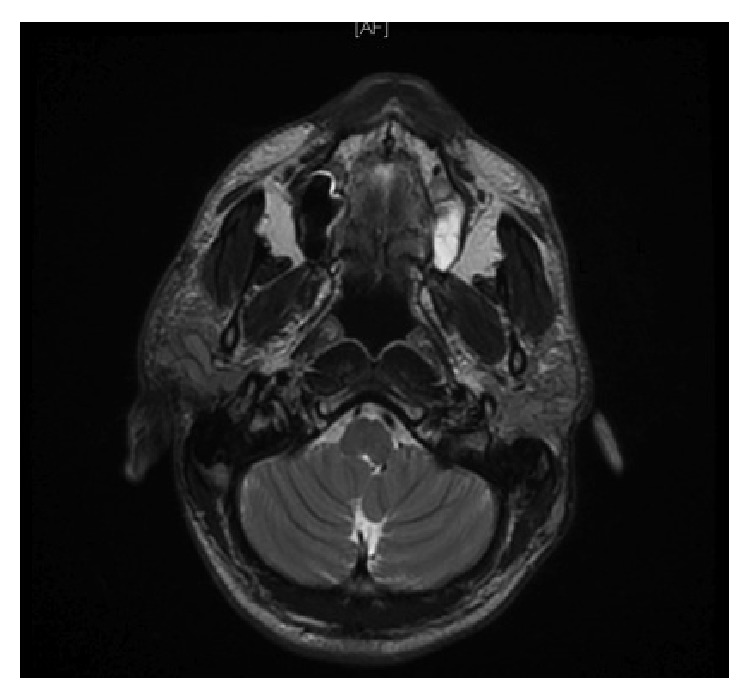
Preoperative MRI T2-weighted axial cut: bilateral hyperintense enlarged parotids, with thickened overlying subcutaneous tissue.
